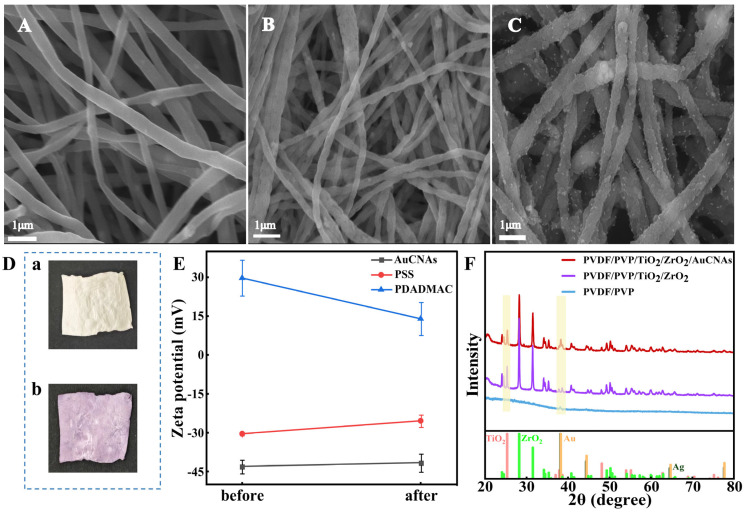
(A–C) SEM images of nanofiber membranes before and after ultrasound and after assembly; (D) nanofiber membrane without AuCNAs loading (a), nanofiber membrane after Au loading (b); (E) zeta potential characterization of AuCNAs sol, PDADMAC, and PSS; (F) XRD images of three nanofiber membranes with different compositions.
